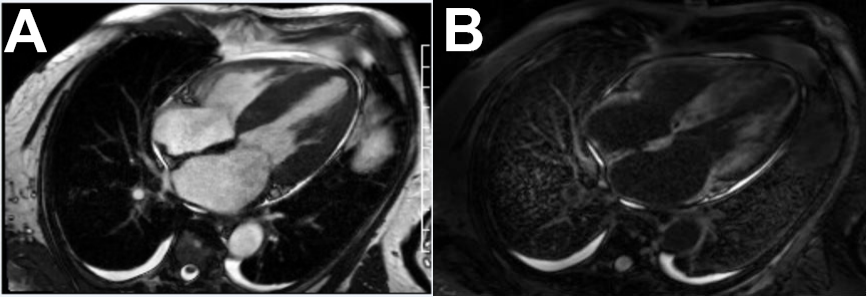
Multimodality cardiac MRI. (A) SSFP standstill showing severe
